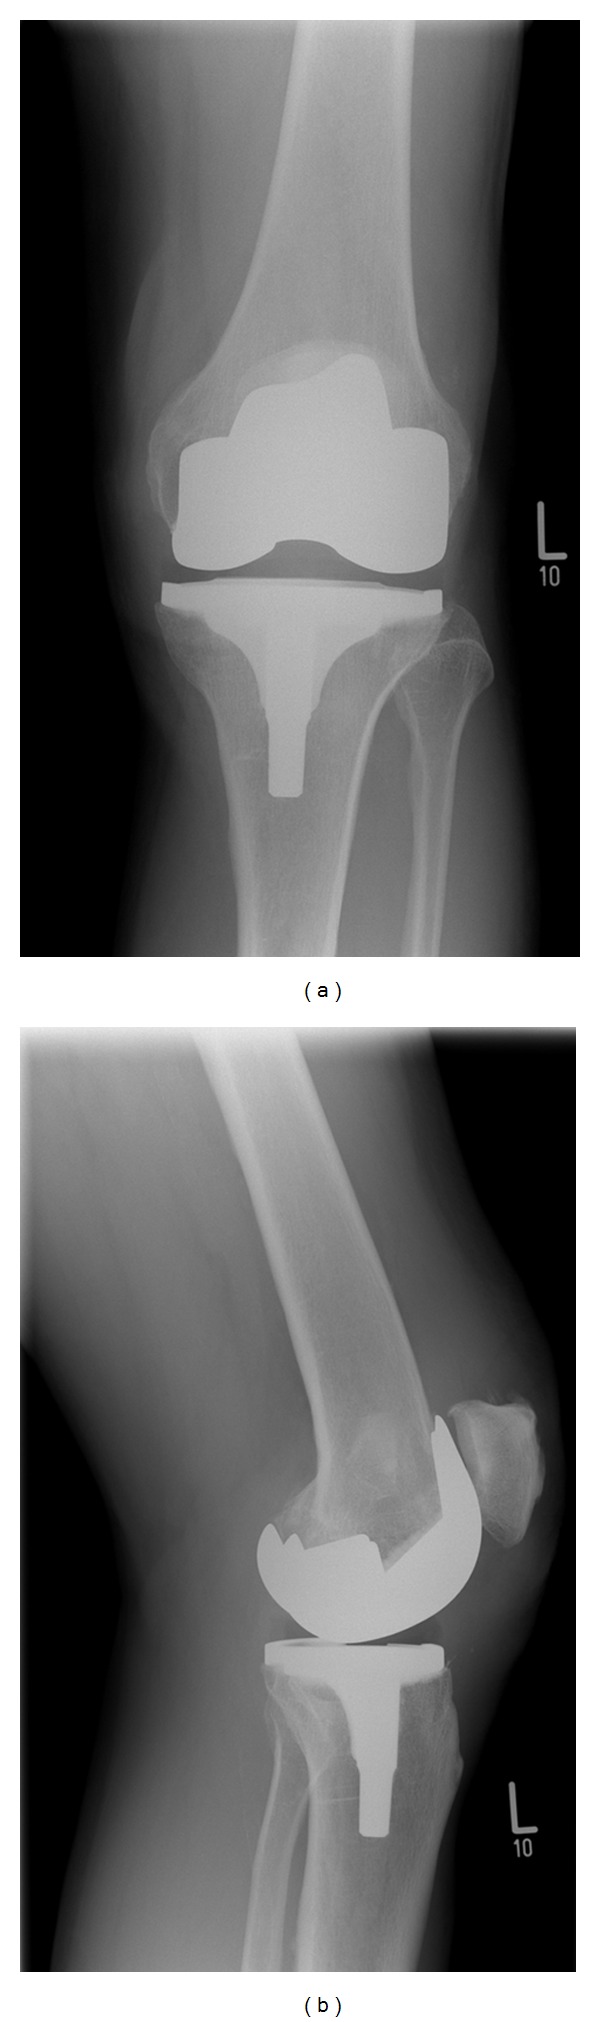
(a, b) Postoperative AP and lateral weight-bearing radiographs showing satisfactory alignment of the prosthesis.
